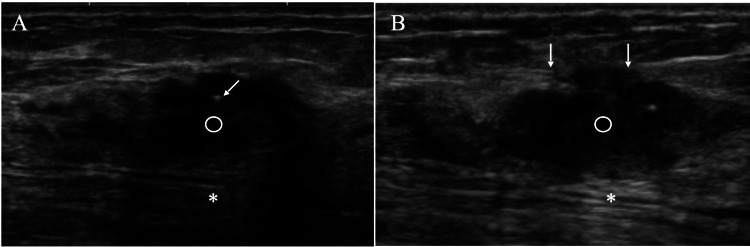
Ultrasound findings A. Ultrasound showed ill-defined low echoic areas (open circle) with an echogenic spot (arrow) and slightly attenuated posterior echoes (asterisk). B. Ultrasound showed no increase in size of the low echoic areas, disruption of the anterior margins of the mammary gland (arrows), and enhanced posterior echoes (asterisk).
